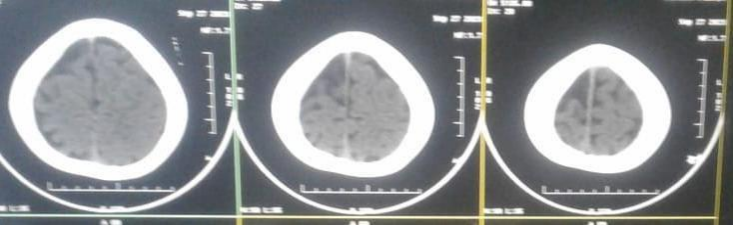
Subtle subcortical calcification in the left temporo-occipital and right parieto-occipital region, without any volume loss on CT brain
